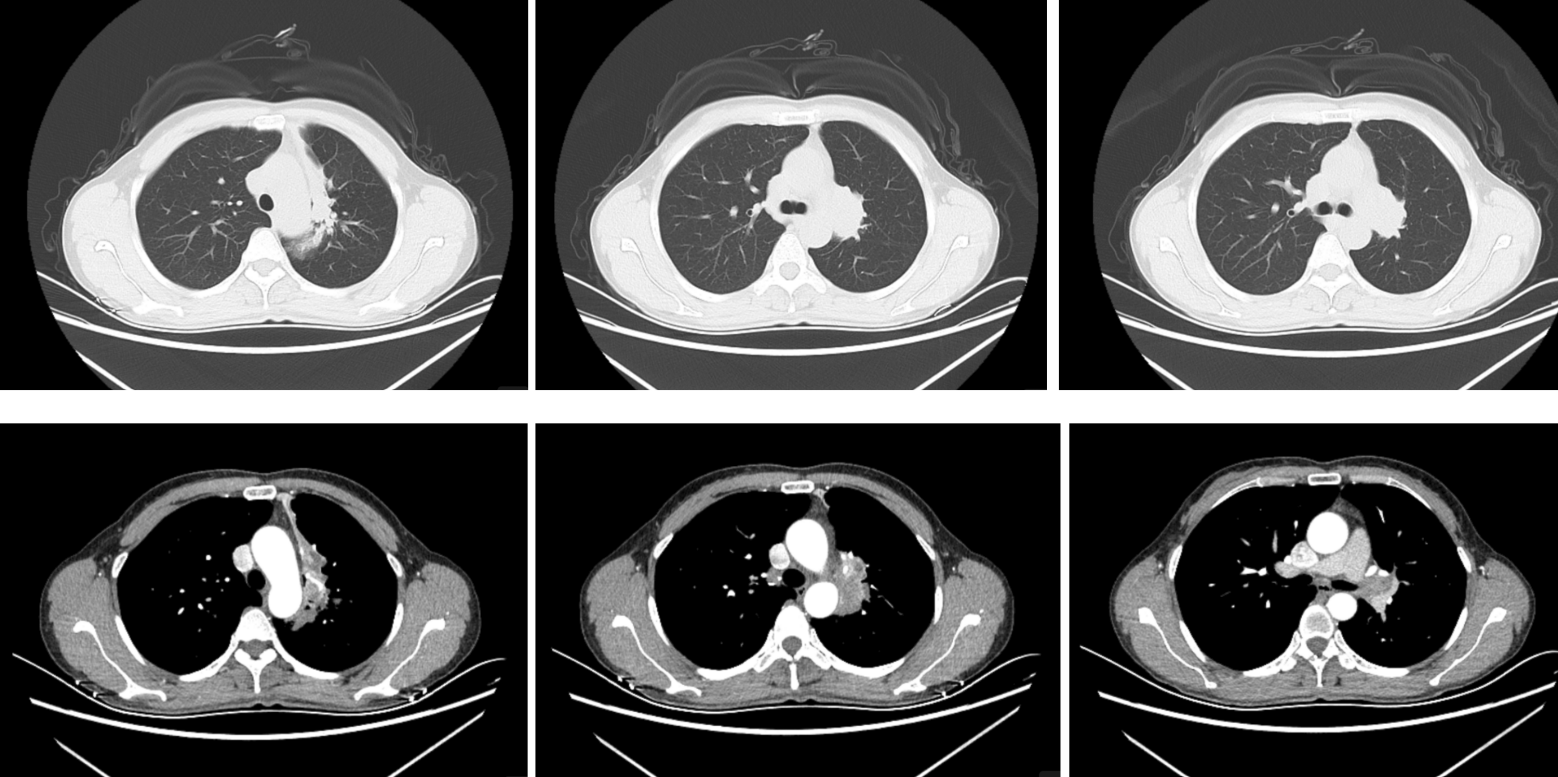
CT of chest showed a hyperenhancing mass in the left upper lobe.
